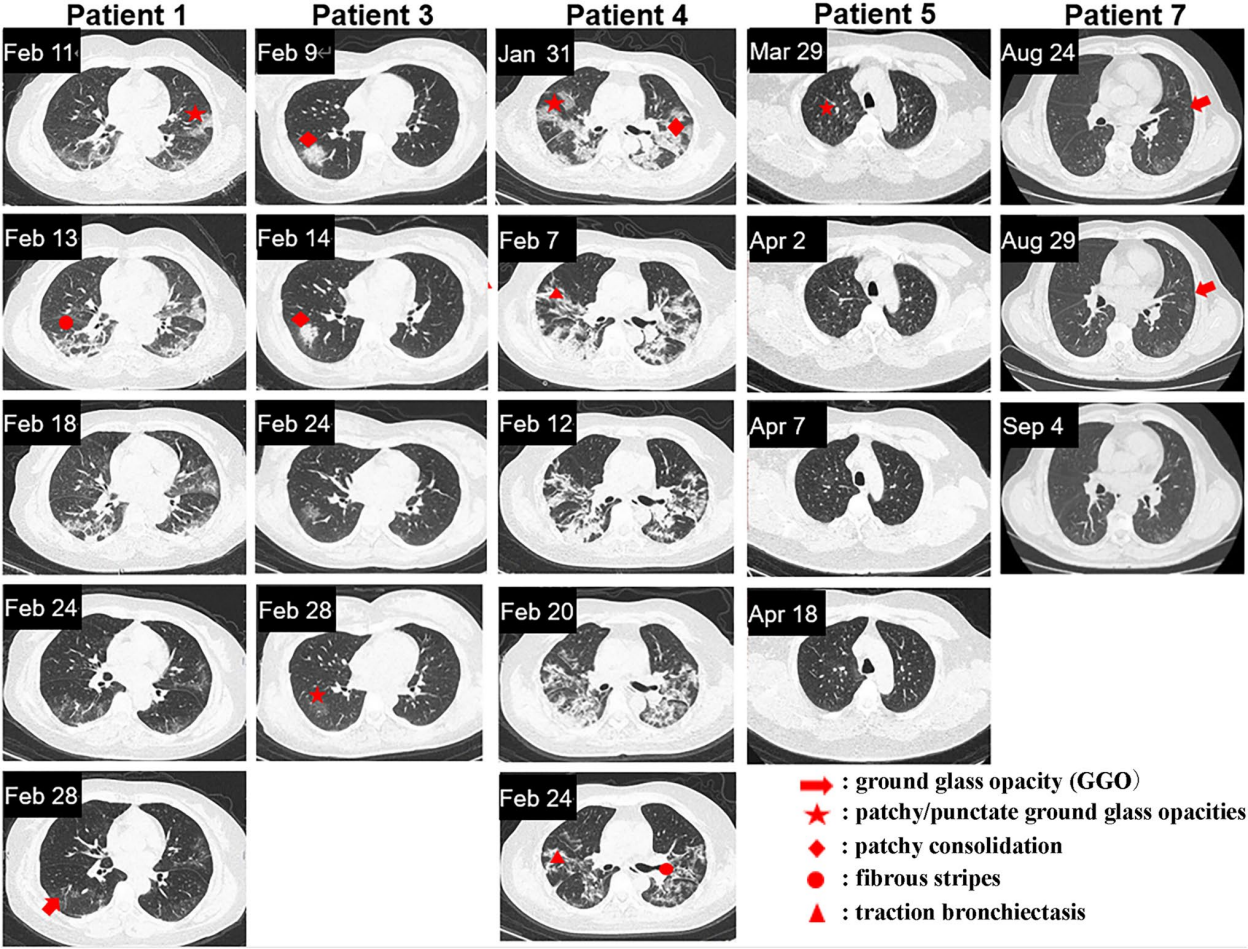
Chest CT images of patients 1, 3, 4, 5 and 7. The exosome treatments for patients 1, 3, and 4 started on February 27, 2020. The exosome treatments for patients 5 and 7 started on April 1, 2020 and August 20, 2020, respectively
